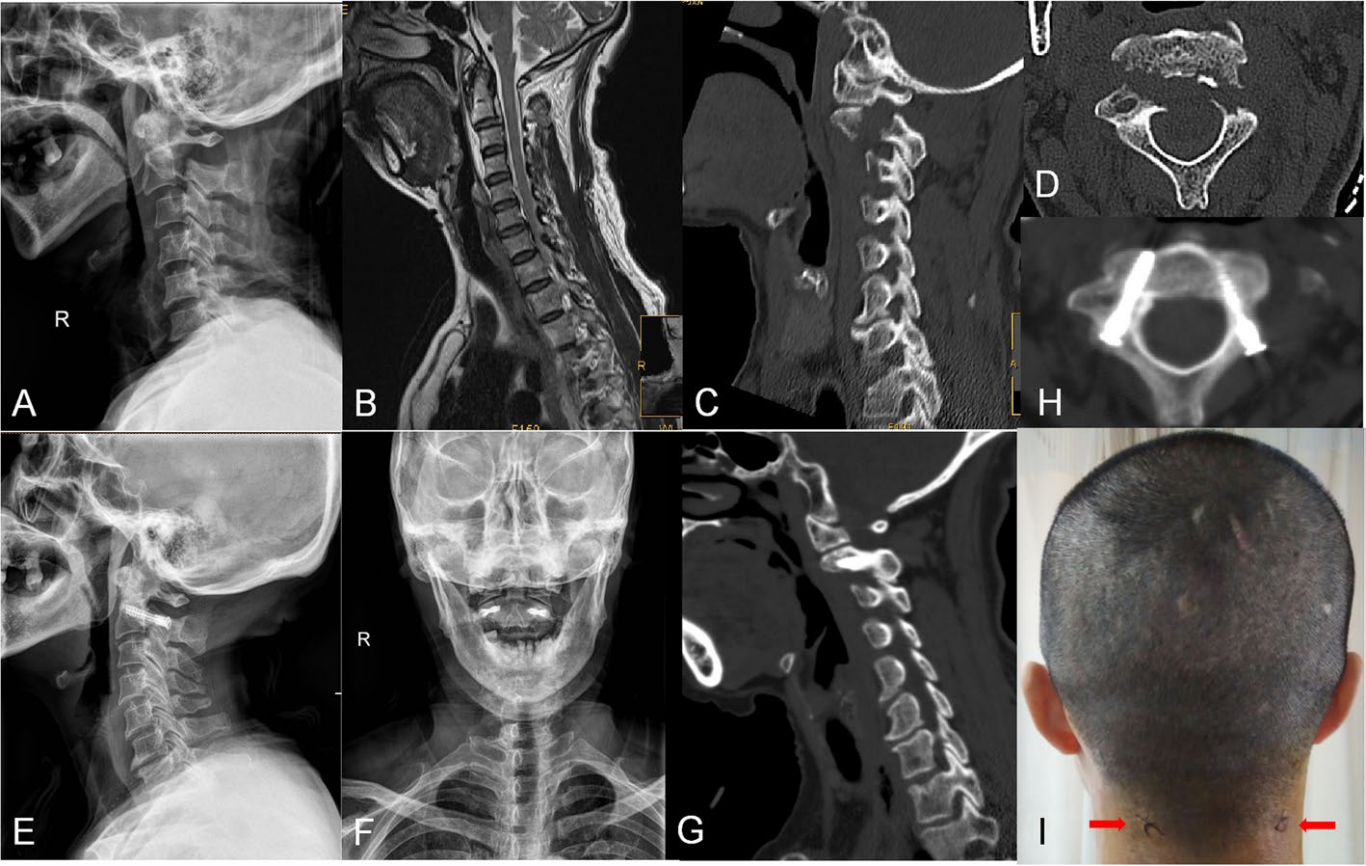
The pre-operative lateral radiographs of a 35-year-old male patient with a type II Hangman fracture (A). Pre-operative sagittal T2-weighted magnetic resonance image revealed the absence of spinal cord compression (B). Pre-operative computed tomography (CT) scan reconstructions show obvious separation of fracture on both the sides(C, D). The post-operative open-mouth and lateral radiographs showed adequate fracture reduction (E, F). The computed tomography (CT) scan reconstructions at the 3-month follow-up showed excellent fusion (G, H). The minimal incisions of this technology (I)
